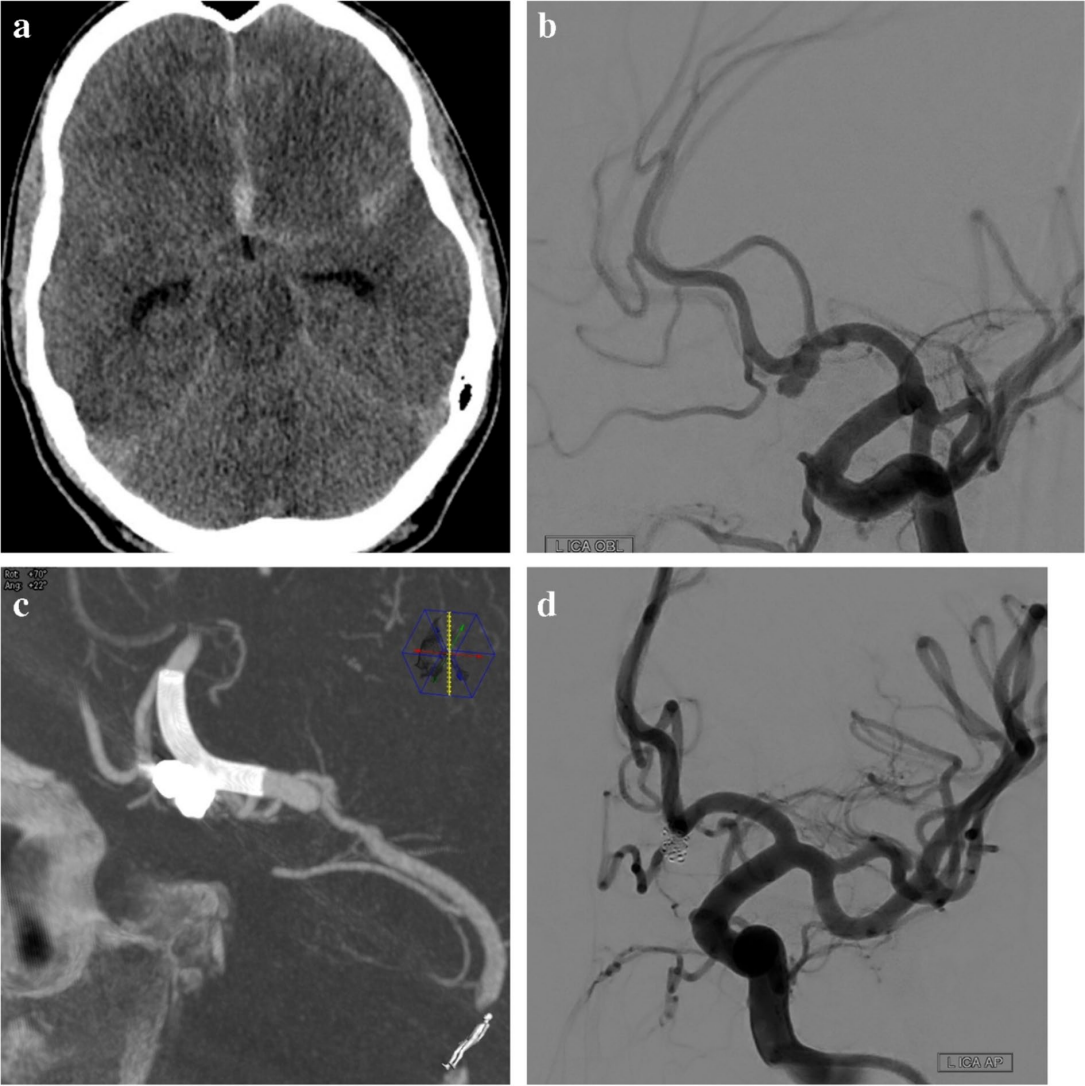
A 24-year-old male patient with subarachnoid hemorrhage (SAH). (a) CT demonstrating SAH predominantly in the anterior interhemispheric sulcus. (b) Left carotid angiogram in a left oblique projection revealing a multilobulated saccular aneurysm at the anterior communicating artery. (c) Cone-beam CT after embolization showing the multilobulated aneurysm filled with coils and the SVB stent positioned in the A1 and A2 segments of the ipsilateral ACA. (d) Twelve-month follow-up angiogram confirms occlusion of the aneurysm
